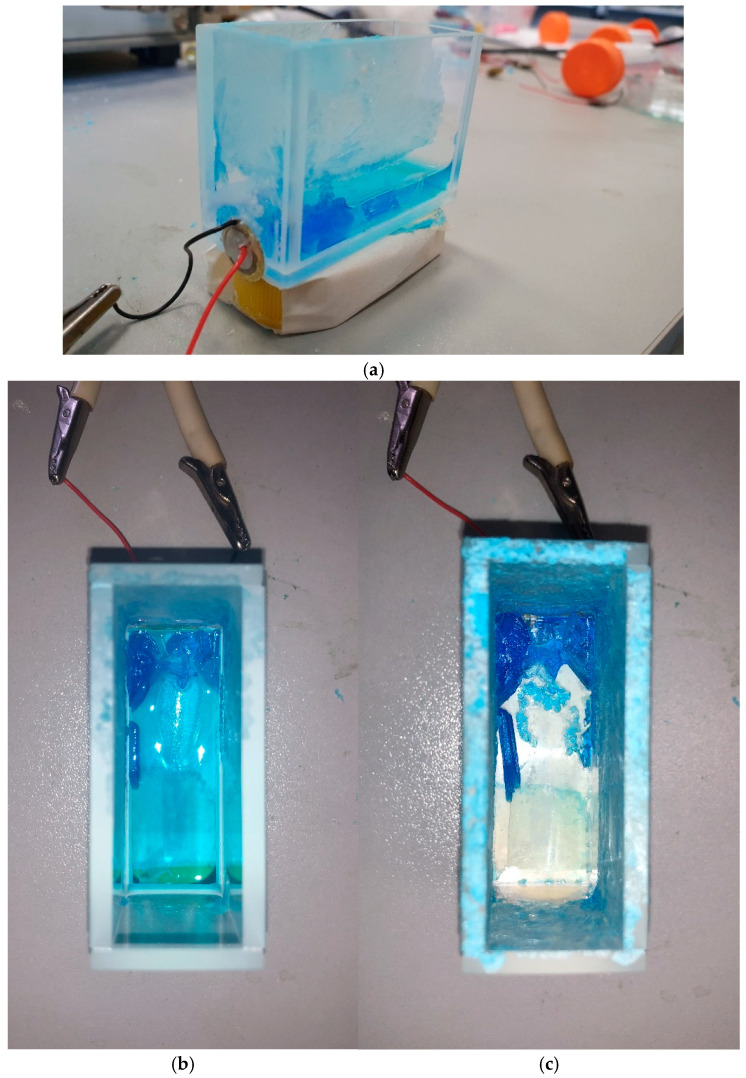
CuSO_4_ crystal hydrate formed on the Nafion substrate from NW-solution under the irradiation with one ultrasonic beam at a pulse voltage of *U*_0_ = 6 V. Panels (a,b): the formation of the deposit continues, since the solution has not completely evaporated. Panel (c): the end of the formation of the deposit; water is absent in the cuvette. It is seen that the deposit is formed predominantly near the piezoelectric transducer.
